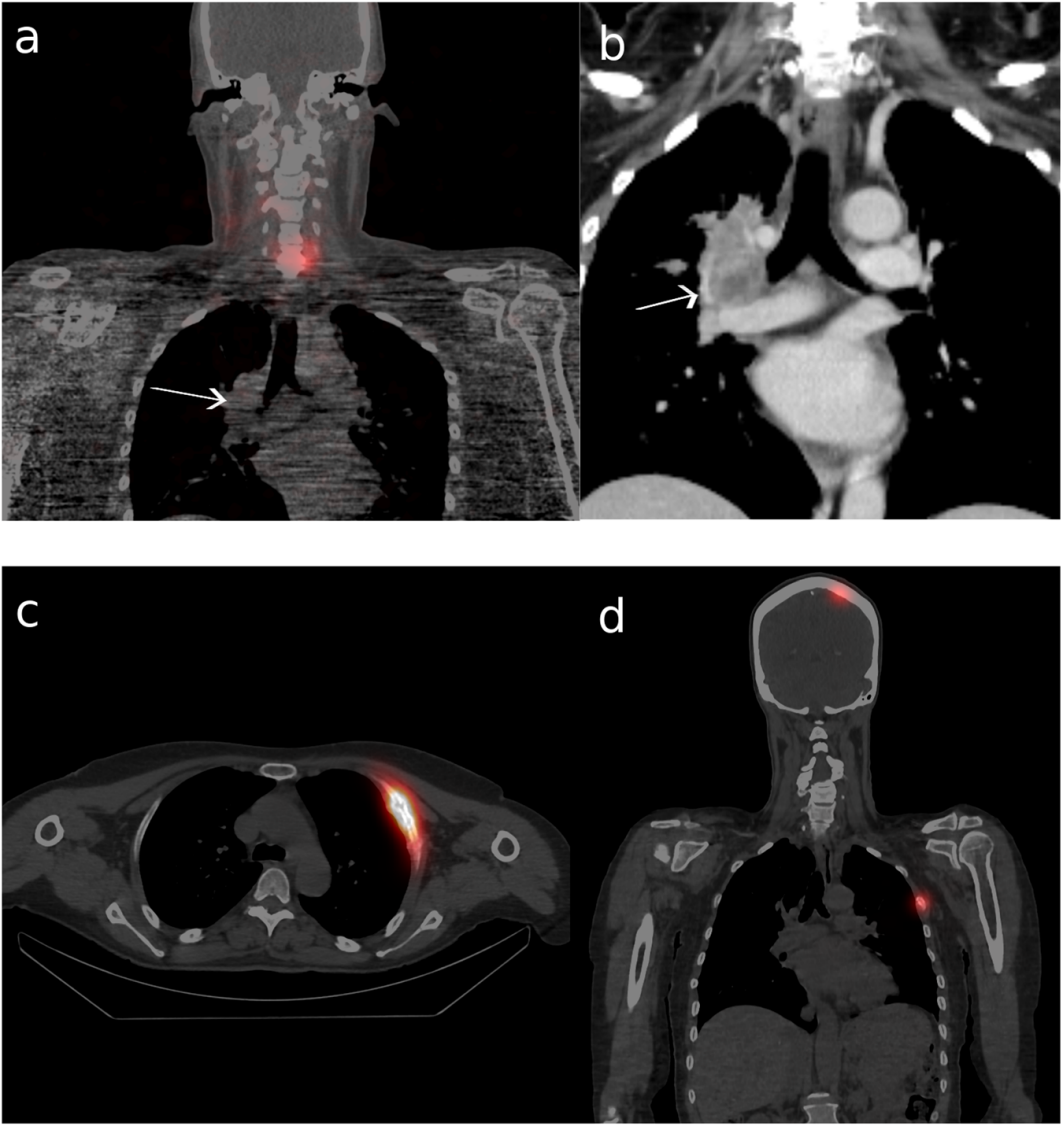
a SPECT/CT and (b) contrast-enhanced diagnostic CT image (taken 1.5 months after the SPECT/CT in (a)) of #2 showing a bronchial metastasis with no visible iodine uptake. Note iodine uptake in remnant tissue on the neck for reference. c Transversal and (d) coronal SPECT/CT image of #9, showing high iodine uptake in metastatic lesions in the skull and rib
